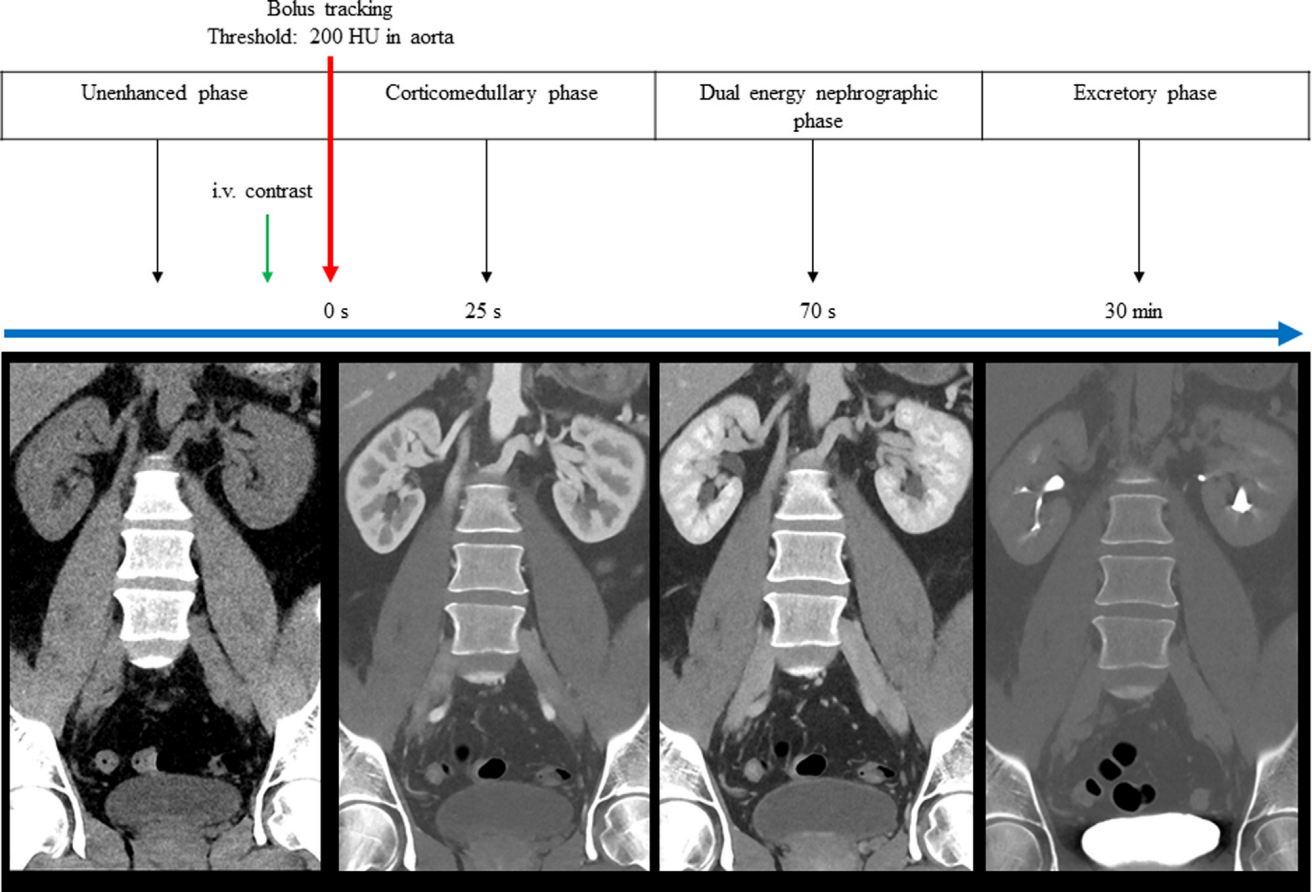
The CT protocol. All patients were asked to drink 1 l of water and not to void urine within 1 h before the CT. The contrast phases were acquired using bolus tracking with a 200 HU threshold in the abdominal aorta. The corticomedullary phase was performed at 25 s and the nephrographic phase at 70 s after the threshold. After the nephrographic phase was completed, patients got off the CT table and were asked to void urine. The excretory phase was performed at 30 min after the threshold. CT = computed tomography; HU = Hounsfield units; i.v. = intravenous.
